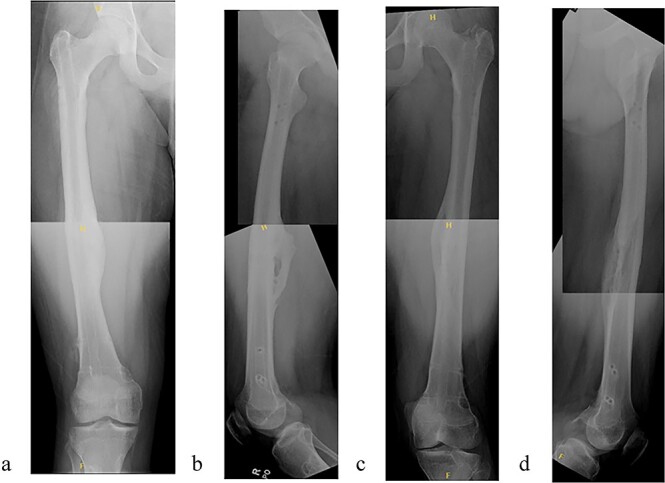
(a) Right femur AP radiograph after implant removal. (b) Right femur lateral radiograph after implant removal. (c) Left femur AP radiograph after implant removal. (d) Left femur lateral radiograph after implant removal.
